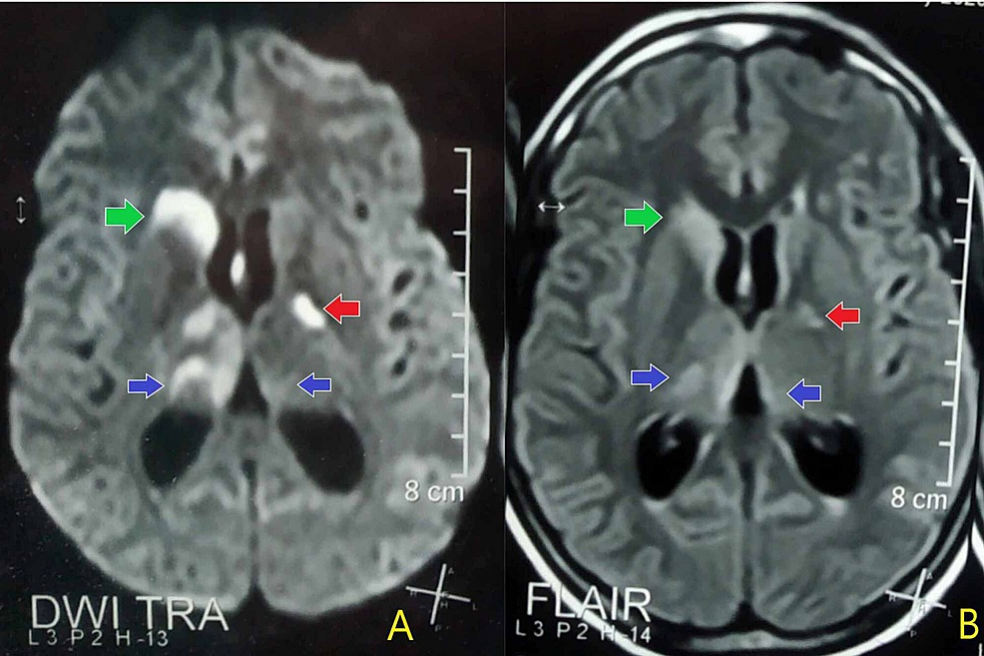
Diffusion restriction in DWI (A) in bilateral thalami (blue arrows), right caudate nucleus (green arrows) and left globus pallidus (red arrows) along with hyperintensity of these regions in (FLAIR) images (B) on MRI brain. DWI: Diffusion-weighted imaging FLAIR: Fluid-attenuated inversion recovery MRI: Magnetic resonance imaging
